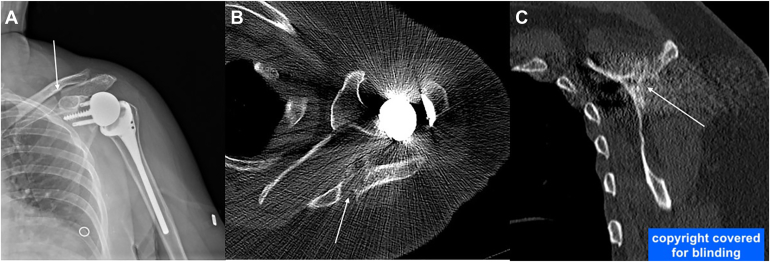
Pre-operative imaging. (A) Grashey view X-ray of the acromion fracture 4 weeks after injury, demonstrating the rTSA to be in adequate position. (B) Coronal view CT scan demonstrating the acromion fracture and rTSA 16 weeks after injury. (C) Sagittal view CT scan 16 weeks after injury. *rTSA*, reverse shoulder arthroplasty; *CT*, computed tomography.
